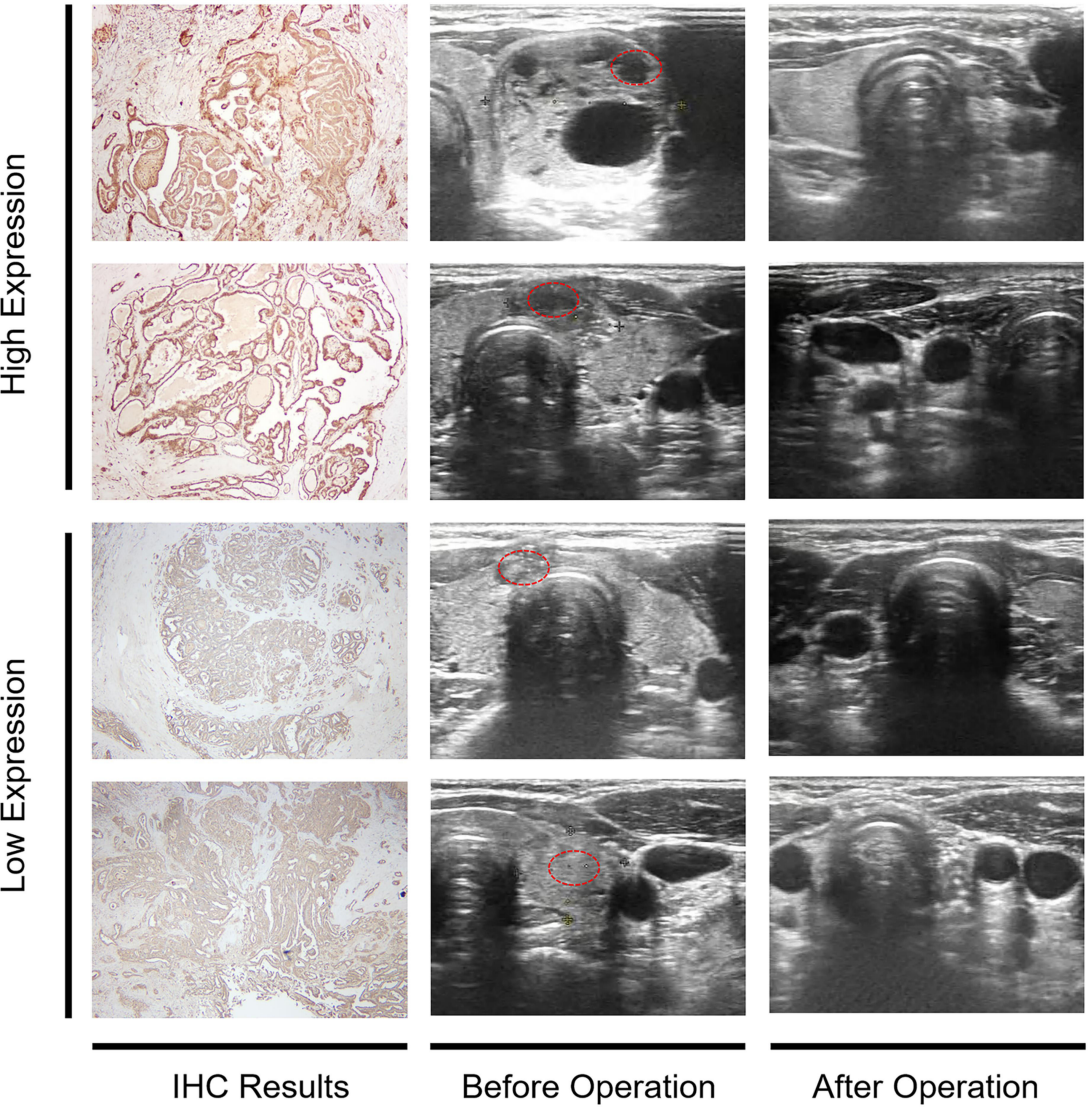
IHC analysis of MYCBP2 expression and clinical follow up of TC patients based on their MYCBP2 expression status.Here, we show four representive IHC results of patients and corresponding ultrasound follow-up image.
